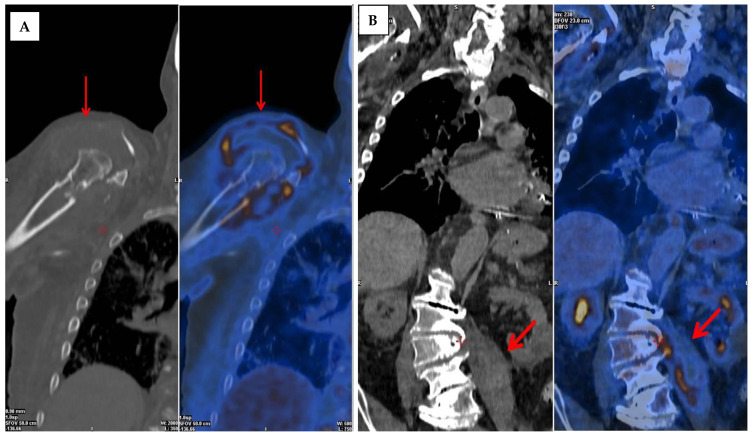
Spondylodiscitis at L2–L4 level and septic arthritis of both shoulders secondary to infective endocarditis due to methicillin-resistant *Staphylococcus aureus*. 18F-FDG PET/CT in 85-year-old male patient with lumbar spondylodiscitis (L2–L4) and septic arthritis of both shoulders, with bacteremia secondary to infective endocarditis on prosthetic aortic valve due to methicillin-resistant *Staphylococcus aureus*. The scan showed a significant FDG uptake in both glenohumeral joints (A) and at the level of the left psoas (B) in relation to a significant abscess with associated L2–L3 spondylodiscitis. Furthermore, elevated focal uptake was found in the aortic valve, compatible with suspected endocarditis (red arrows).
